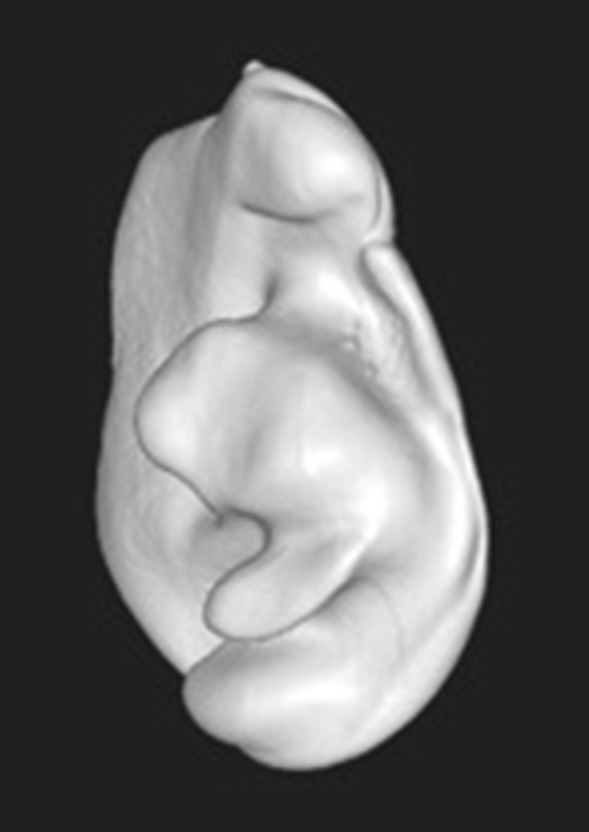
Converting the impression to a virtual three-dimensional data set that can be imported to planning software. In this case, a CBCT-scan was used
